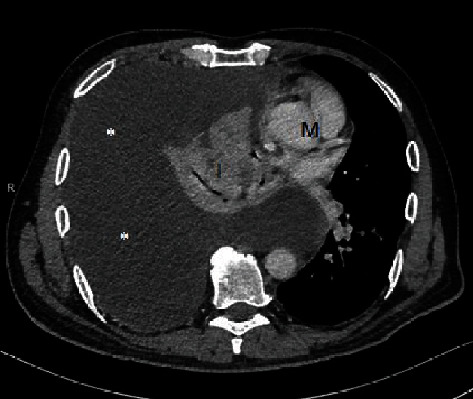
Computed tomography of chest cavity after intravenous contrast injection in axial plane. Free fluid in the right pleurum with atelectasis of the right lung and mediastinal shift. ^∗^Free fluid in pleural cavity. L: lung; M: mediastinum.
